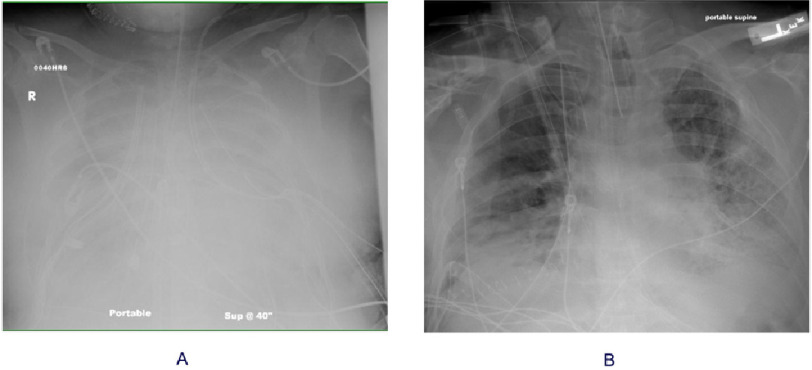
Representative chest radiographs of primary graft dysfunction. Panel A: Fulminant pulmonary edema 48 h after bilateral lung transplant for severe idiopathic pulmonary arterial hypertension and right heart failure. PaO_2_:FiO_2_ ratio < 100. Procedure performed with cardiopulmonary bypass and required large volume blood transfusion. Died on postoperative day 7. Panel B: Patchy right lung opacities consistent with pulmonary edema 72 h after right single lung transplant for idiopathic pulmonary fibrosis. PaO_2_:FiO_2_ ratio: 184. Procedure done without extra-corporeal support or blood transfusion. No pre-transplant pulmonary hypertension. Donor had active smoking and vaping history. Recipient duration of mechanical ventilation was 22 days. At 17 months follow-up, has stage I chronic lung allograft dysfunction, restrictive phenotype with pleural fibrosis.
